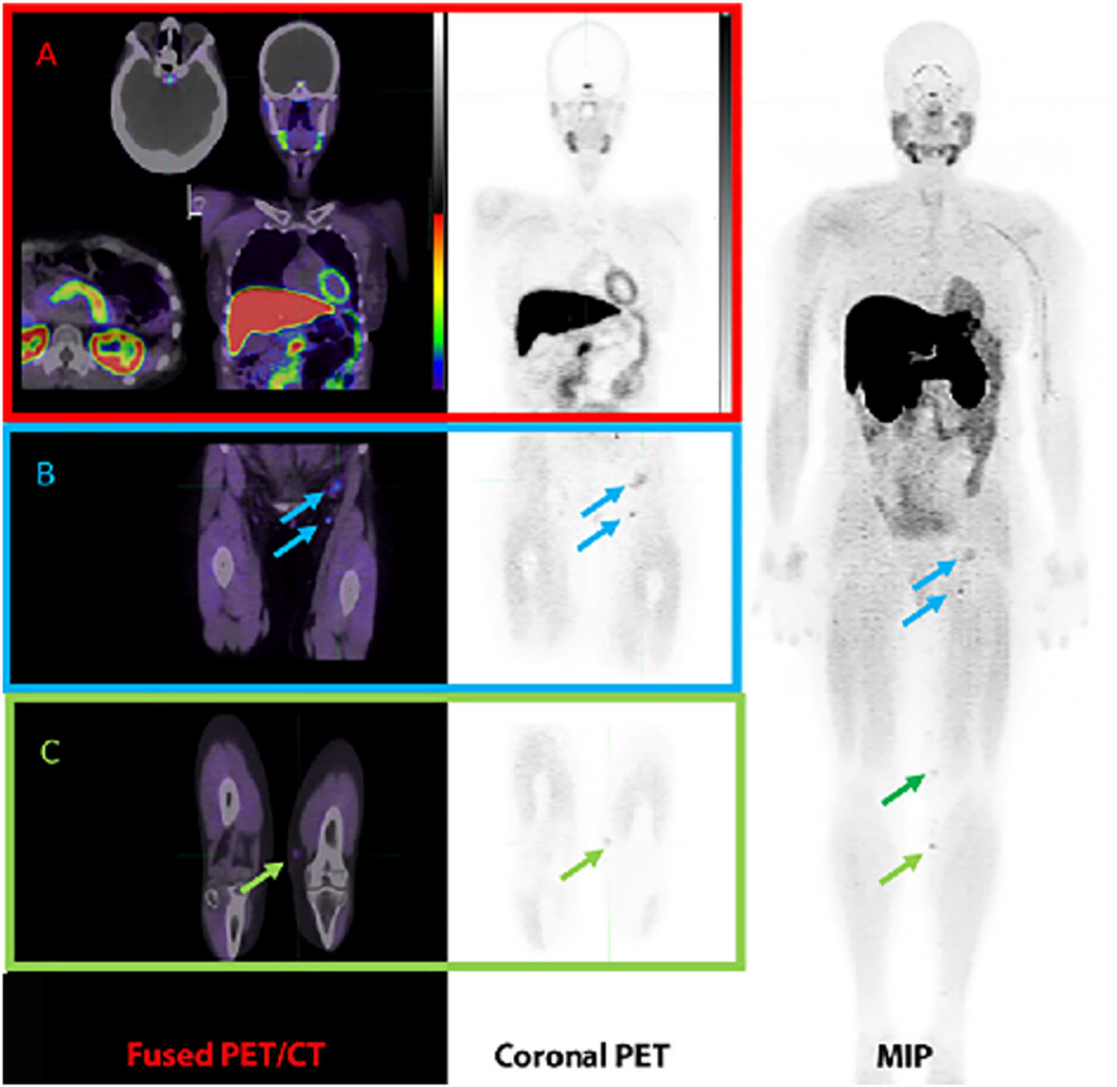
Imaging activated CD8 T cells with ^18^F-AraG. Baseline imaging a patient with recurrent malignant melanoma, scanned at 60 min after IV injection of approximately 185 MBq of ^18^F-AraG prior to immune check-point therapy. A Maximum intensity projection (MIP) image demonstrates uptake in inguinal nodal disease (Blue arrows) and in transit nodules (Green arrows). Panel (A): Fused PET/CT and coronal PET images demonstrate moderately intense uptake in the pituitary, salivary glands, myocardium, liver and pancreas. All these organs are subject to immune-related adverse events and how this biodistribution will impact diagnostic performance is unclear. Panel (B): Increased uptake is observed in two inguinal lymph nodes, with associated nodal enlargement of the upper node. Panel (C): The small lower in transit nodule is clearly identified on both PET and correlative CT (Image provided by Dr Diwakar Davar and Dr Shyam Srinivas from University of Pennsylvania Medical Center and Dr Jelena Levi of CellSight).
